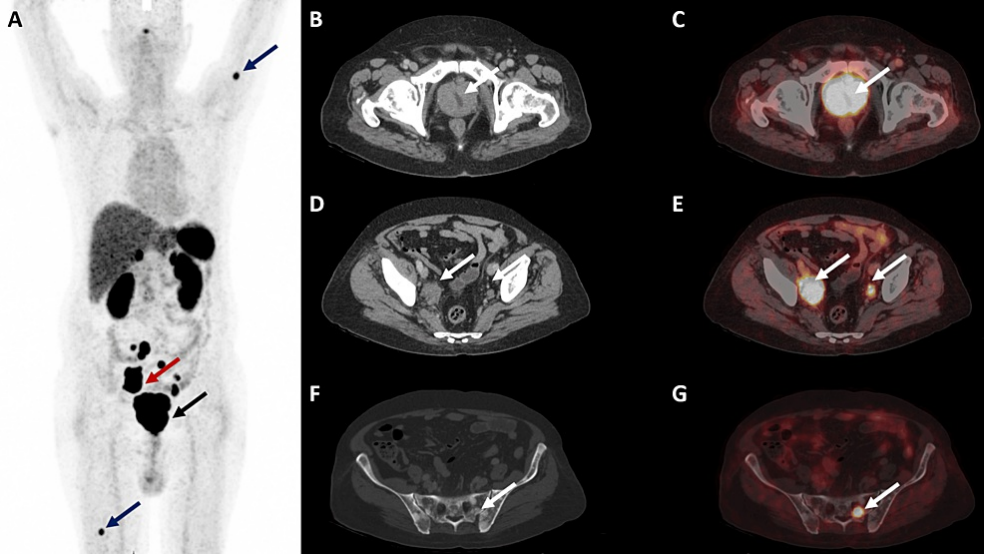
68Ga - DOTANOC PET/CT images of the patient (A) MIP; (B, D, & F) axial view of CT; (C, E, & G) axial view of 68Ga - DOTANOC PET/CT fusion. Image A shows DOTANOC uptake in the region of the prostate (black arrow), pelvic lymph nodes (red arrow), and skeletal metastatic sites in the region of the left humerus and right femur (blue arrows). Images B & C reveal prostate mass with DOTANOC uptake suggesting somatostatin receptor-expressing neoplastic disease (white arrows). Images D & E show bilateral iliac lymph nodes with DOTANOC uptake (white arrows). Images F & G show a sclerotic lesion involving the sacrum with DOTANOC uptake (white arrows). MIP: Maximal intensity projection; 68Ga-DOTANOC PET/CT: 68Gallium-labeled DOTANOC positron emission tomography/computed tomography.
